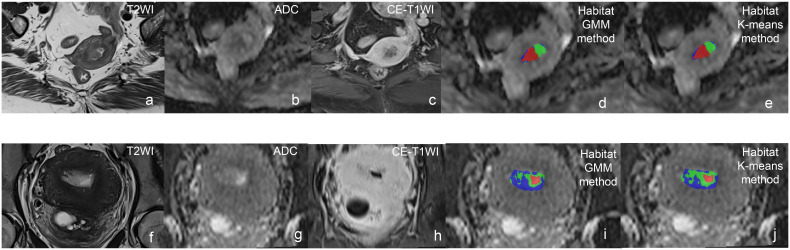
MRI habitat imaging. Case 1: A 63-year-old woman with endometrial cancer (a–e). The red areas represent hypervascular cellular tissue. The green areas represent hypovascular cellular tissue. The blue areas represent non-solid components. Case 2: A 49-year-old woman with endometrial hyperplasia (f–j).
